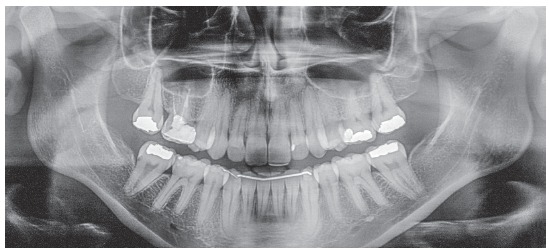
Panoramic radiograph nine years after treatment completion.
